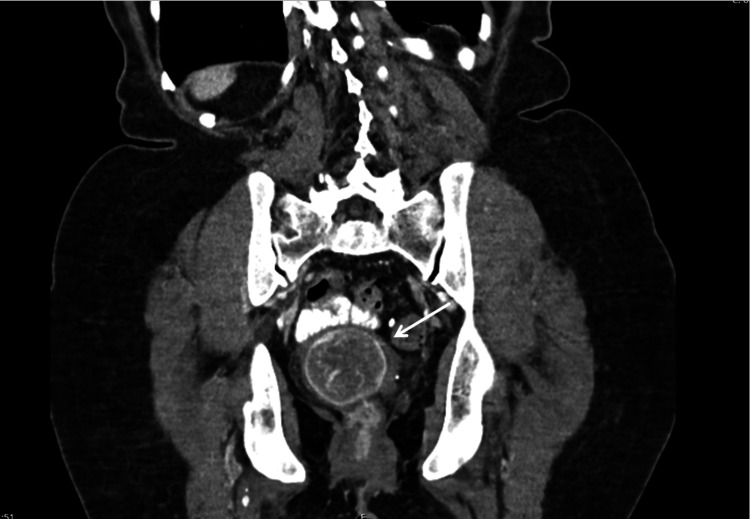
Abdominopelvic computed tomography. The uterus is indicated by the white arrow.
